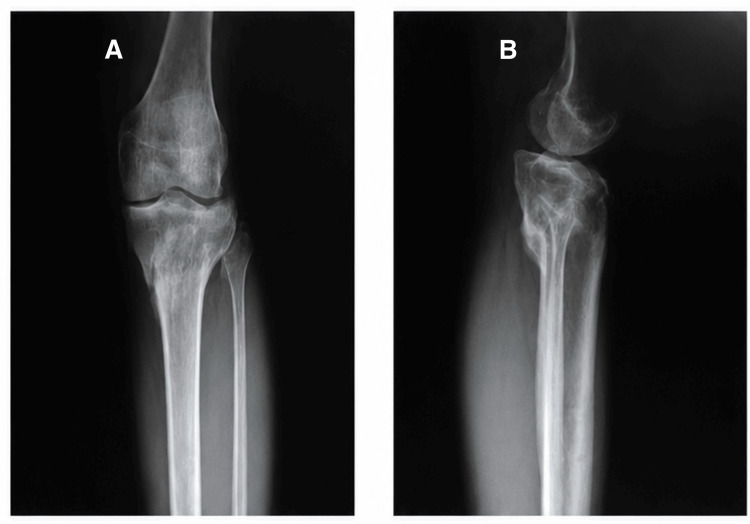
Four-month postoperative anteroposterior (A) and lateral (B) radiographs demonstrating satisfactory coronal alignment and bridging callus
